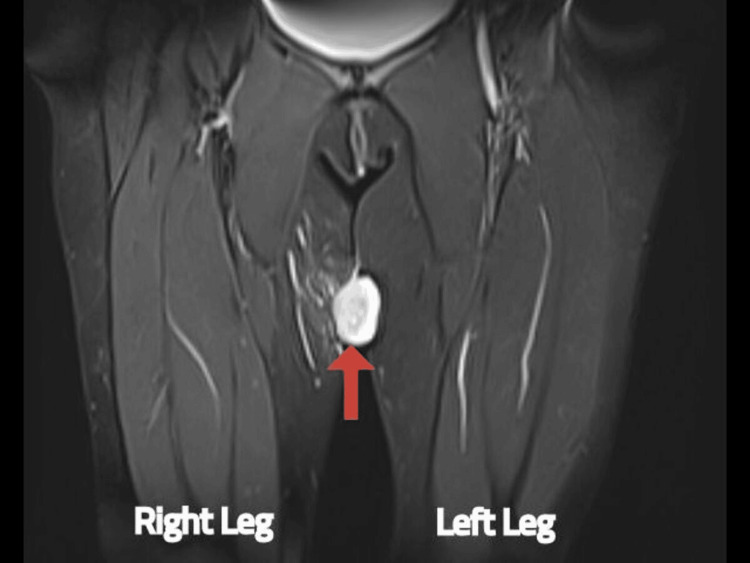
MRI of the proximal thigh shows soft-tissue swelling in the coronal view MRI: magnetic resonance imaging The red arrow shows soft-tissue swelling in the right proximal thigh.
